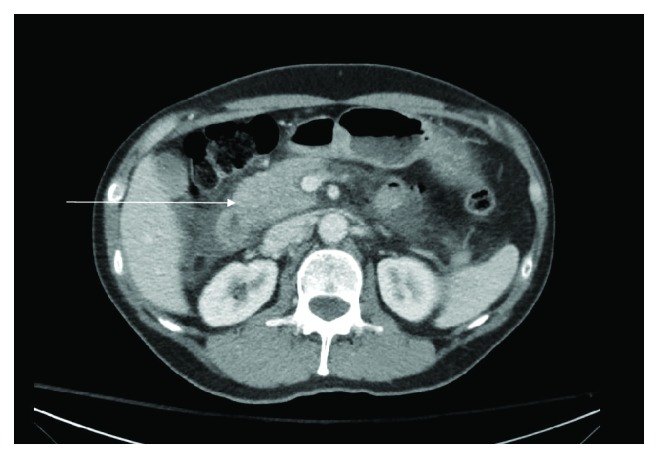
Computed tomographic image of the abdomen showing acute interstitial pancreatitis (arrow) with small discrete fluid collection in the uncinate process. Also noticeable is a moderate amount of inflammatory fluid in the anterior pararenal space and a small amount in the retroperitoneum.
